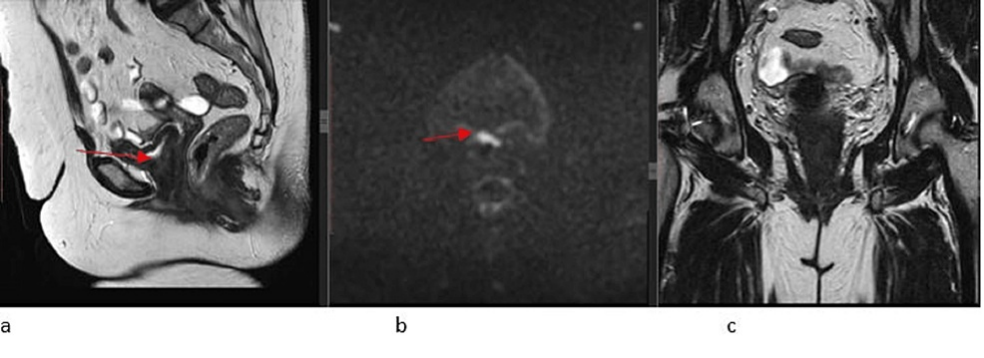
MRI pelvis, post six cycles of chemotherapy shows a good treatment response with a further interval decrease in the abnormal T2 hyperintense signal area involving the upper two-thirds of the vagina on sagittal T2WI as shown by the red arrow (4a), small area of diffusion restriction on DWI (4b) with resolution of pelvic side wall lymphadenopathy (4c); the cervix is clear DWI: diffusion-weighted imaging
